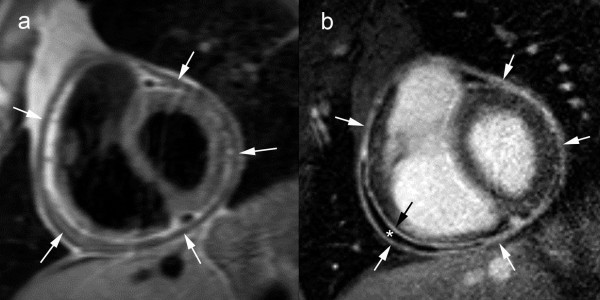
Inflammatory-constrictive form of constrictive pericarditis. Short-axis T1-weighted spin-echo CMR (a) and LGE CMR (b). Diffusely thickened pericardium, with strong enhancement of the pericardial layers after gadolinium administration (arrows). Presence of a small pericardial effusion (*) along the inferolateral side of the right ventricle. Real-time CMR in cardiac short-axis during free breathing shows increased ventricular coupling with inspiratory septal inversion and increased right-sided septal motion at onset of expiration. At surgery, a heavily thickened, inflamed and constrictive pericardium was found.
